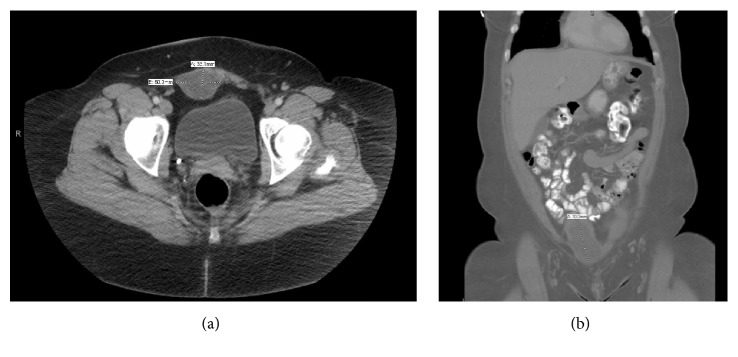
CT image of the abdominal mass shows a right lower rectus muscle heterogeneous collection: axial (a) and coronal (b) views.
